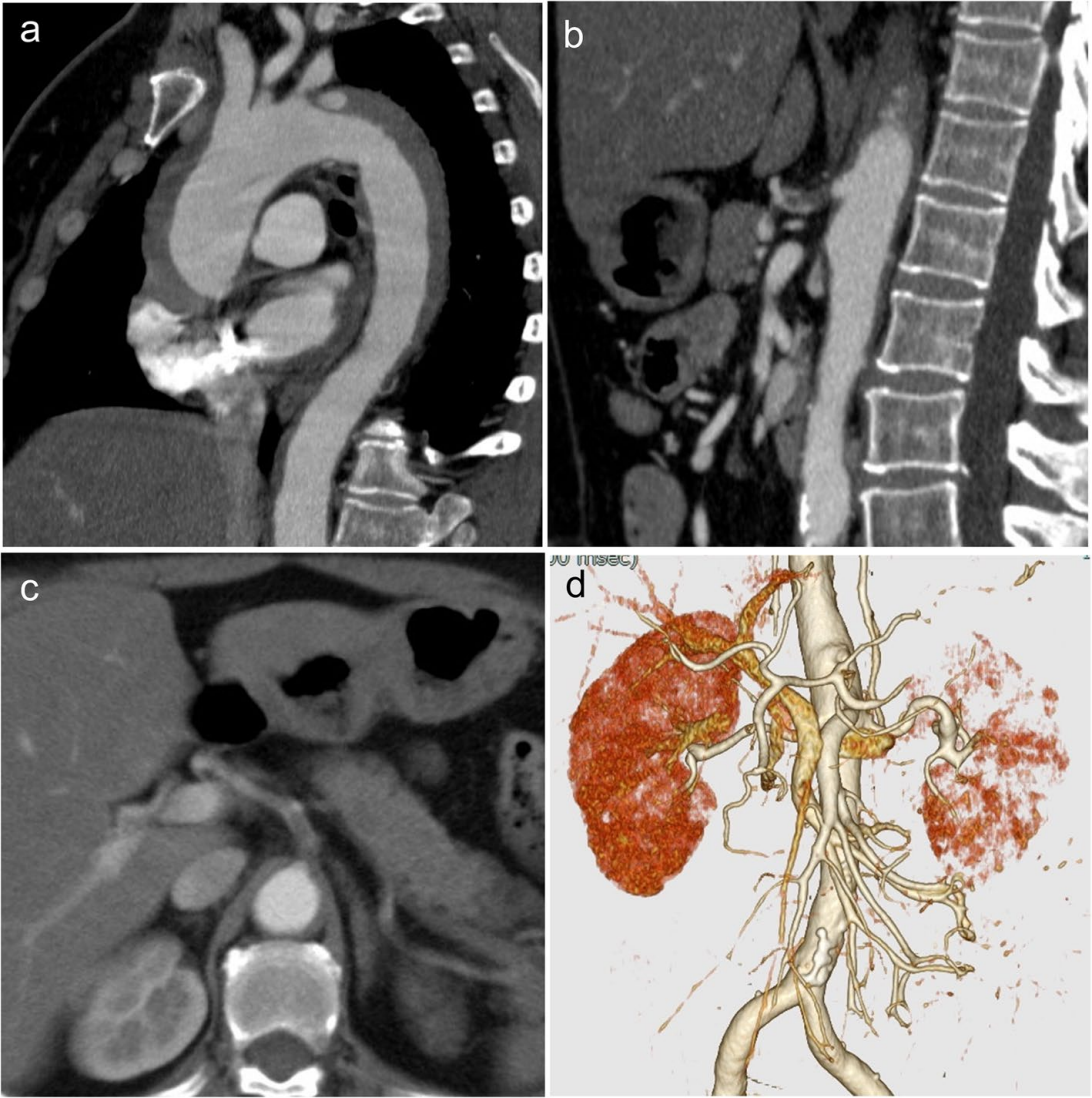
a, b, c, and d Preoperative contrast-enhanced CT. a Thrombosed type A acute aortic dissection, b and c severely stenotic celiac artery, and d poor collateral pathways of the celiac artery and superior mesenteric artery
